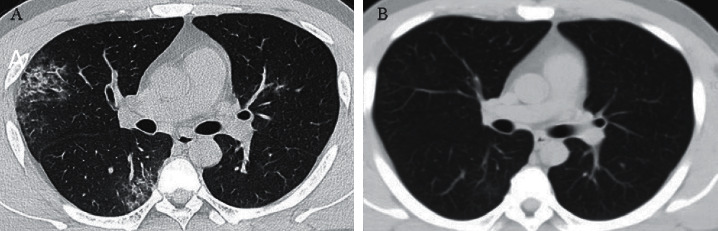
(a) At discharge, lung CT showed multiple patchy and flocculent high-density shadows in both lungs, with blurred edges, especially in the periphery. Some of them showed ground-glass changes and thickened interlobular septa. (b) The lung lesions were absorbed by reexamination three months after discharge.
